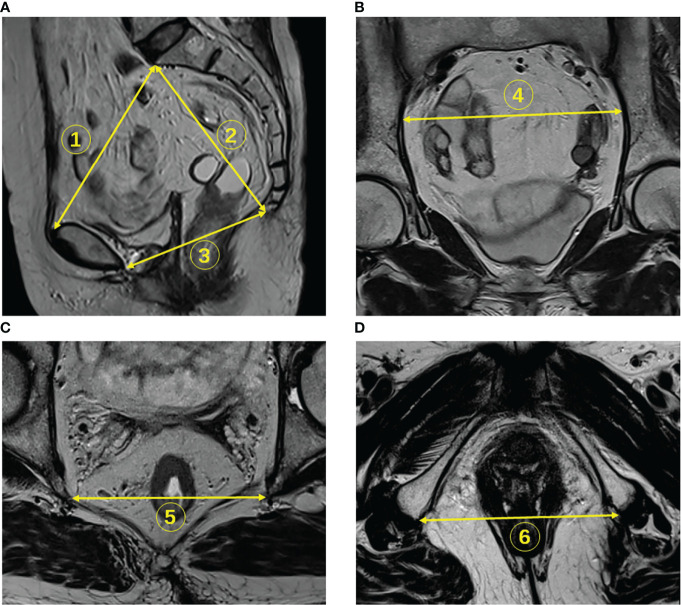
Identification of pelvimetry measurements on MR images. (A) The pelvic inlet (line 1), pelvic depth (line 2), and pelvic outlet (line 3) were measured on sagittal T2WI. (B) The transverse diameter was measured on coronal images (line 4). (C) The interspinous distance was measured on axial T2WI (line 5). (D) Intertuberous distance was measured on axial sections (line 6).
